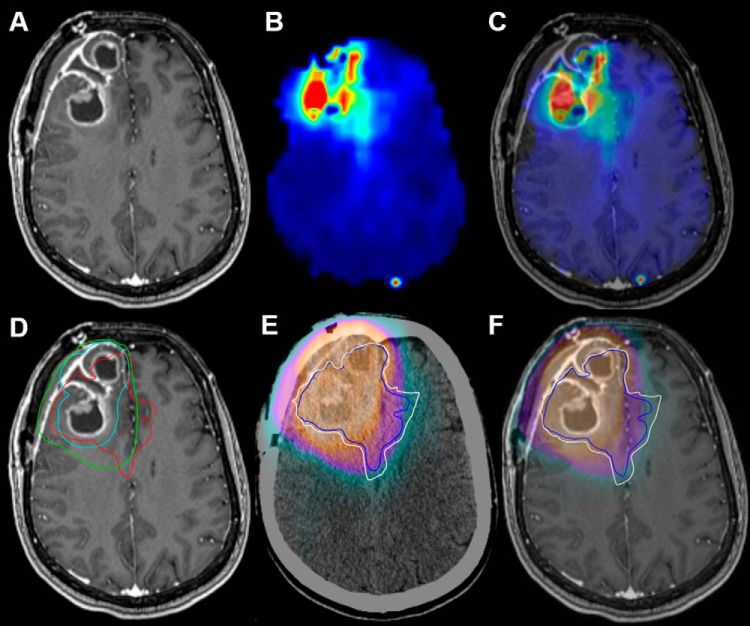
Contrast-enhanced T1-weighted (CE-T1W), choline to N-Acetylaspartate (Cho/NAA) map, and dose fields for a patient with a frontal glioblastoma (GBM) who received standard chemoradiation (patient 3). Cho/NAA abnormalities extended medially and posteriorly from the contrast-enhancing border, implying contralateral infiltration (A–C). A region of 2.0-fold Cho/NAA increase (red contour) falls outside the clinical target volumes (CTVs)2 (turquoise contour) and CTV1 (green contour) near the midline (D). A comparison of the cumulative RT dose with 2.0-fold (blue contour) and 1.75-fold (white contour) Cho/NAA changes shows a large portion of metabolic abnormality falling outside of the region receiving 60 Gy (E and F).
